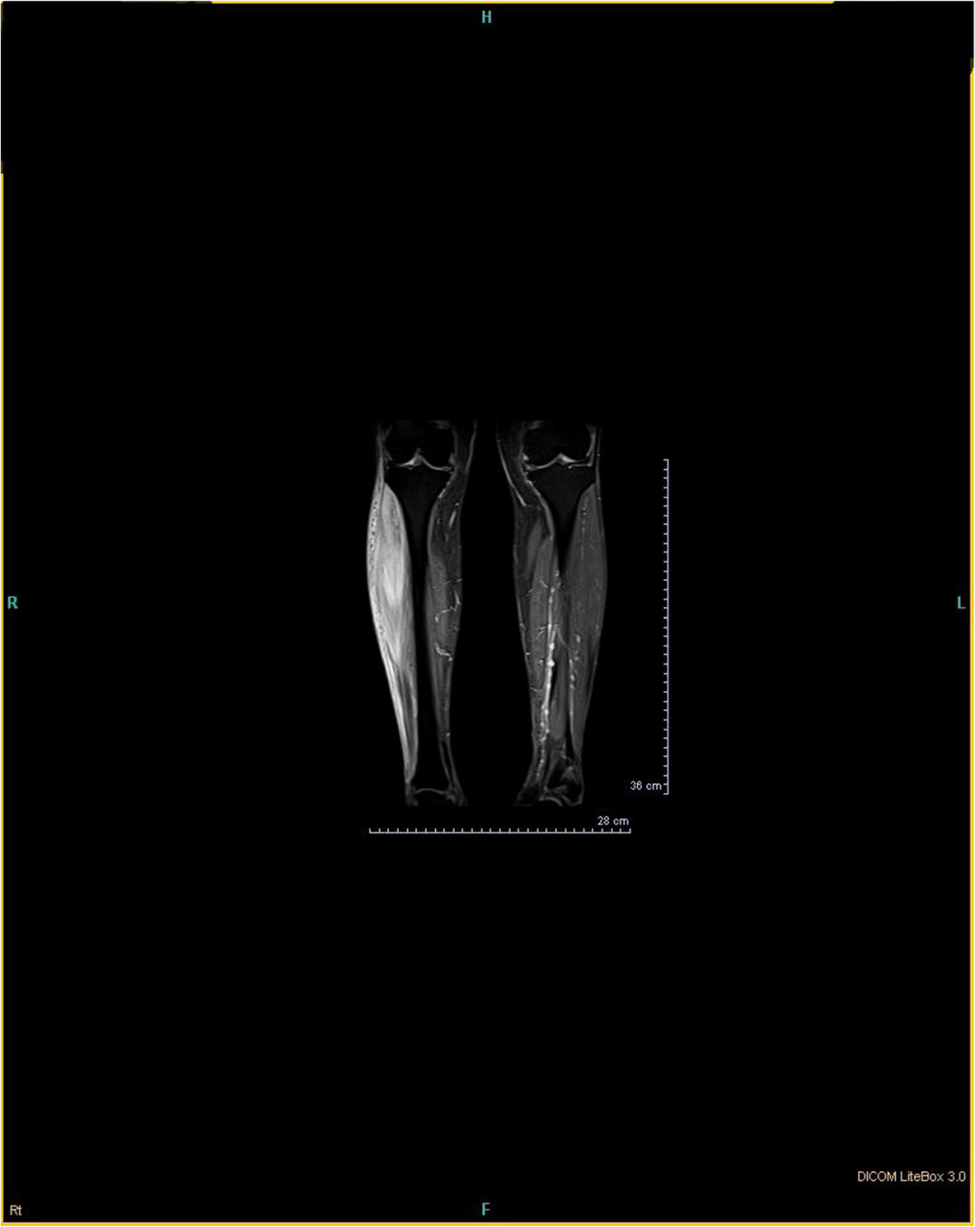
MRI shows a heterogenous enhancement in right tibialis anterior muscle in T2-weighted images.
